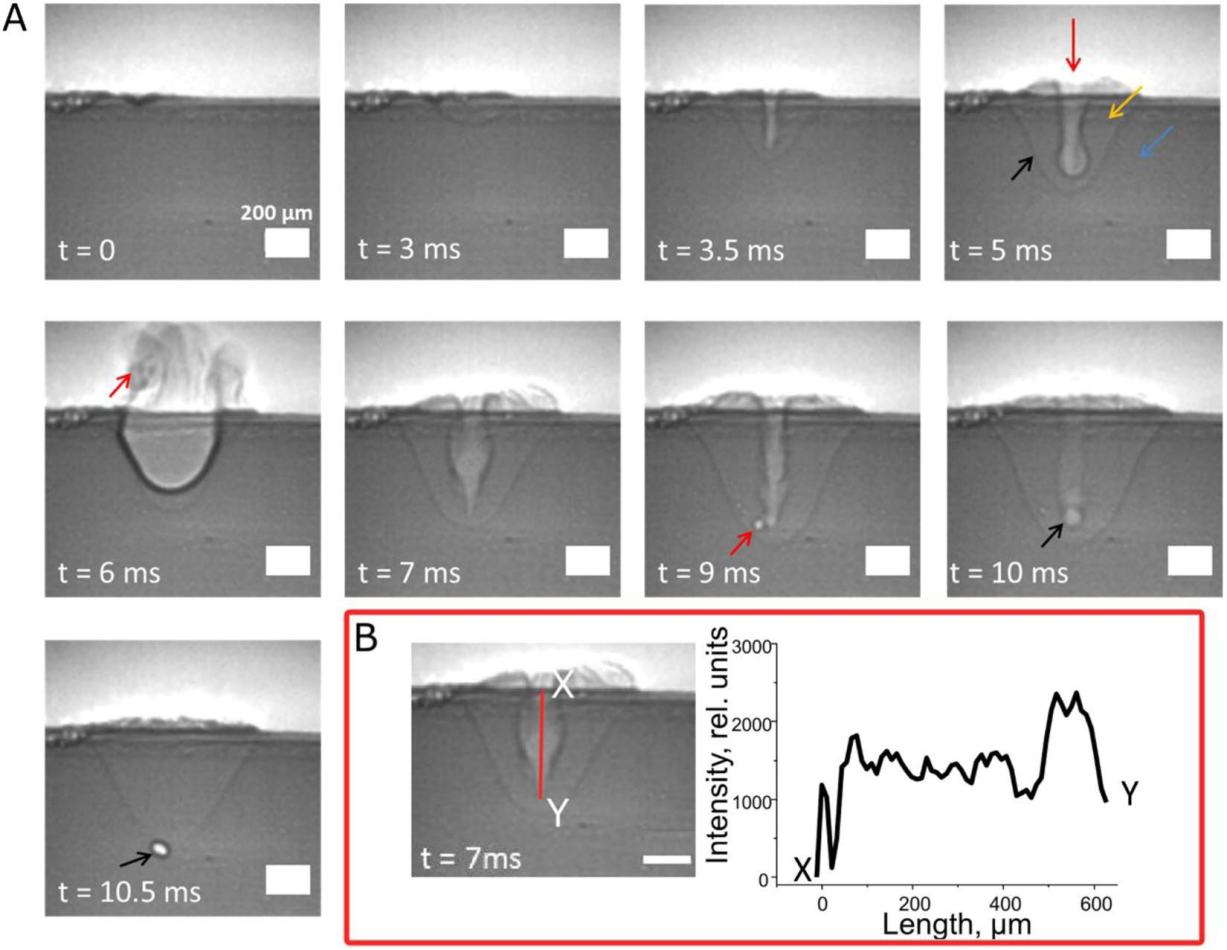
(A) Typical X-ray snapshots of the aluminum sample exposed to a single laser pulse of 10 ms pulse duration at a power of 1 kW. The sample was shifted relatively to the laser beam with a constant velocity of 1.5 mm/s. The time stamps are counted from the start of the laser irradiation. The scale bar at bottom right defines a distance of 200 µm. The red, orange, blue and black arrows at *t* = 5 ms mark the gas phase, liquid phase, solid phase and the liquid/solid boundary, respectively. The red arrows at *t* = 6 ms and *t* = 9 ms show the molten material expelled during blowout and the pore formed during unstable keyhole, respectively. The black arrows at *t* = 10–10.5 ms show the pore formed by the rapid collapse of the keyhole at the end of the irradiation; (B) Pixel intensity profile of the keyhole channel. The brighter pixels correspond to regions with lower density^28^.
